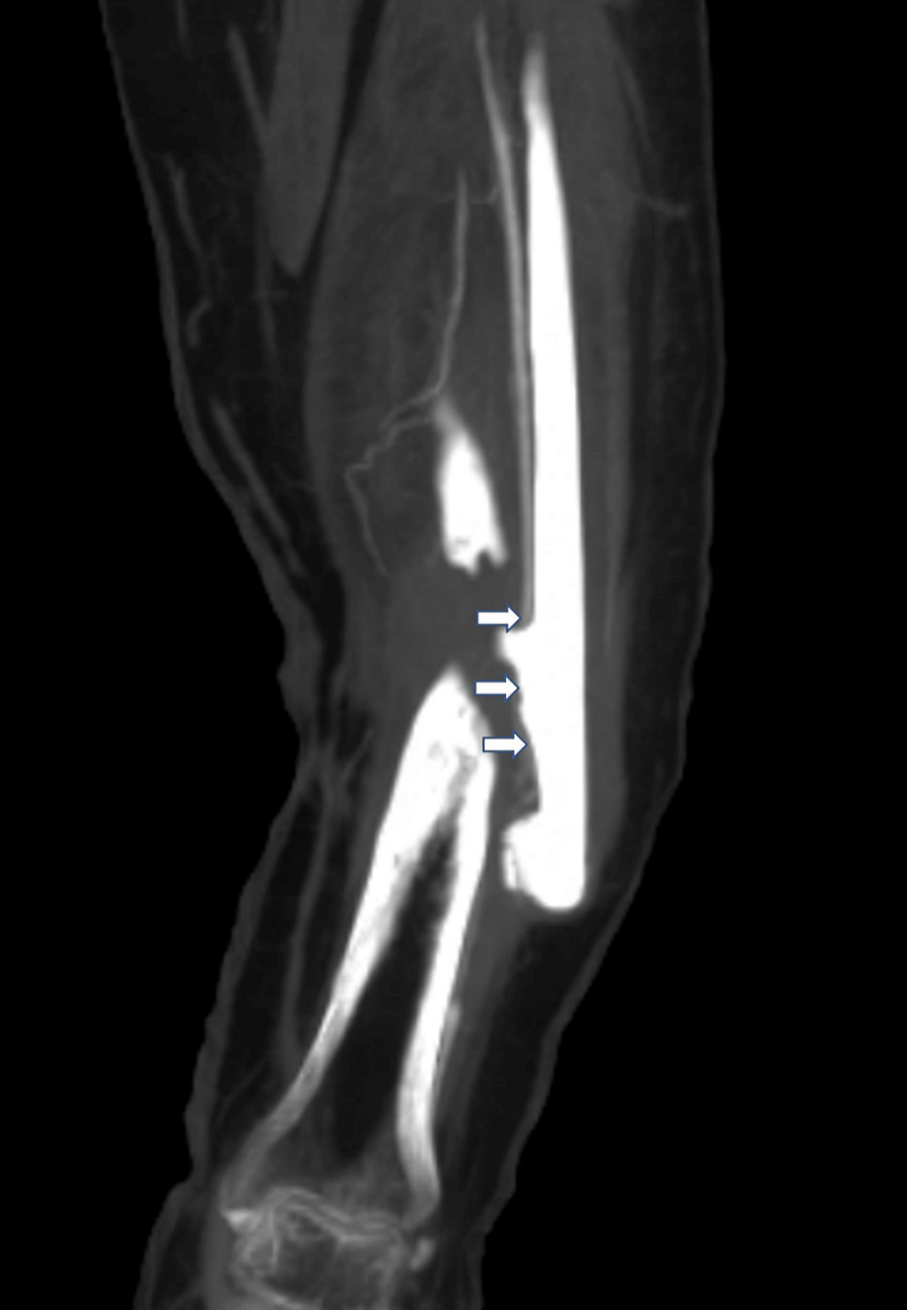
CT angiography revealing occlusion of left anterior tibial artery near the level of defect.
